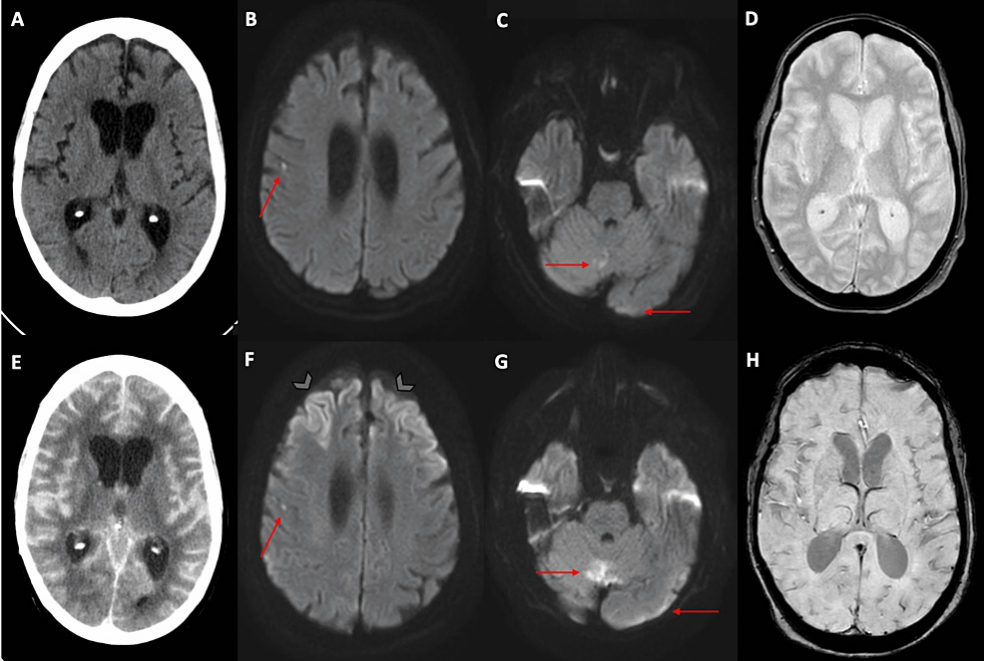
Initial and repeat imaging findings (A) Initial CT (axial section) of the head with contrast showing no subarachnoid hemorrhage. (B, C) Initial MRI of the brain (axial section, diffusion-weighted imaging) showing acute punctate infarct in the right frontal lobe and cerebellum (red arrows). (D) Initial MRI of the brain (susceptibility-weighted imaging) without signs of bleeding in the subarachnoid space. (E) Repeat CT (axial section) of the head with contrast after six hours showing hyperattenuation in subarachnoid space concerning SAH. (F, G) Repeat MRI of the brain (axial section, diffusion-weighted imaging) after six hours showing hyperintensity of bilateral frontal lobes (blue arrowheads) as well as punctate intensities in the right frontal lobe and cerebellum (red arrows). (H) Repeat MRI of the brain (susceptibility-weighted imaging) after six hours showing no dark signal in subarachnoid space to indicate true SAH.
